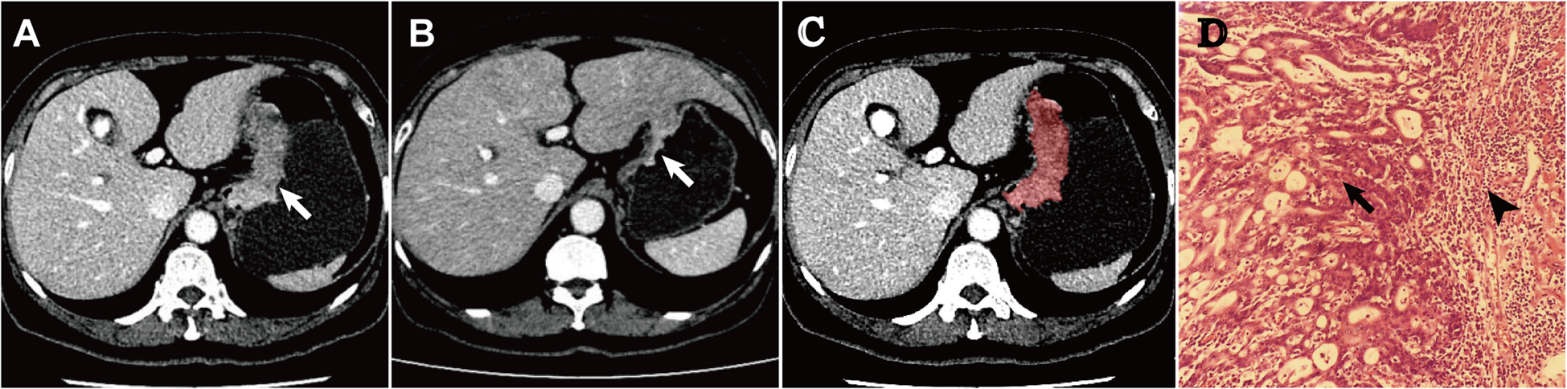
A female patient was diagnosed as gastric cancer (T4aN2M0). CT before neoadjuvant chemotherapy (a) showed a mass-type tumor measured 25 mm in maximal depth and 80 mm in maximal length. CT after neoadjuvant chemotherapy (b) showed a shrunken mass measured 14 mm in depth and 40 mm in length. CT before neoadjuvant chemotherapy (c) showed the ROI delineated manually on figure (a). Pathology examination after surgery (d) showed residual tumor tissue (arrow) and infiltrated inflammatory cells (arrow head)
